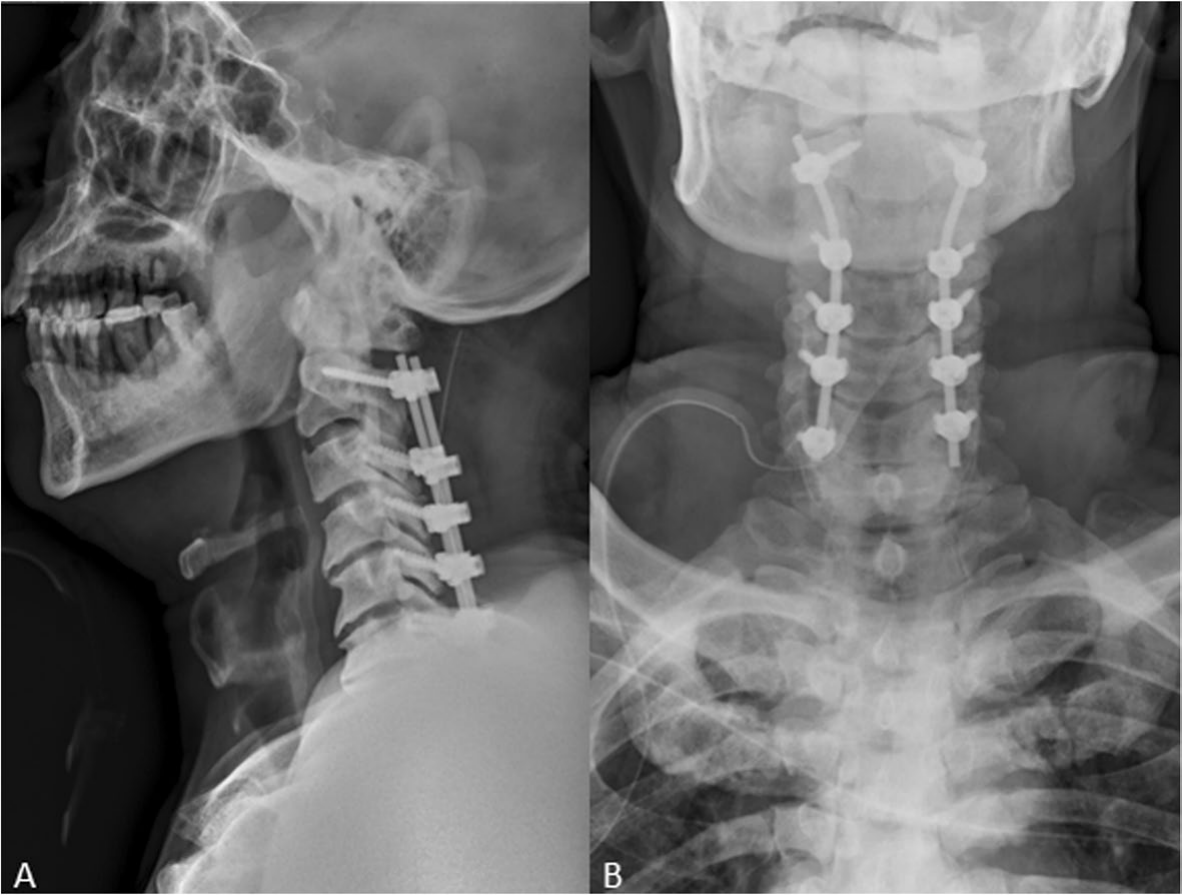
A Lateral and B anterior X-rays show that pedicle screws were inserted into C2
